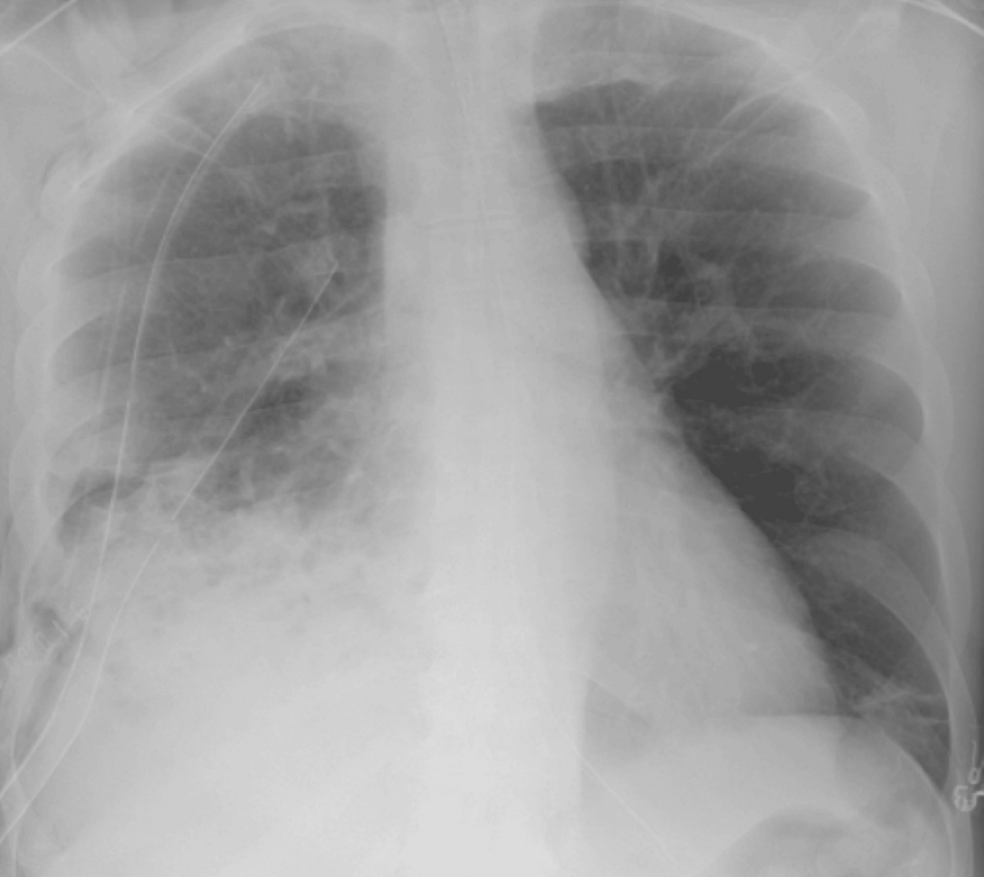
Post-operative AP chest X-ray showing improvement in the right-sided opacities and blunting of the right costophrenic angle. AP: Anteroposterior
